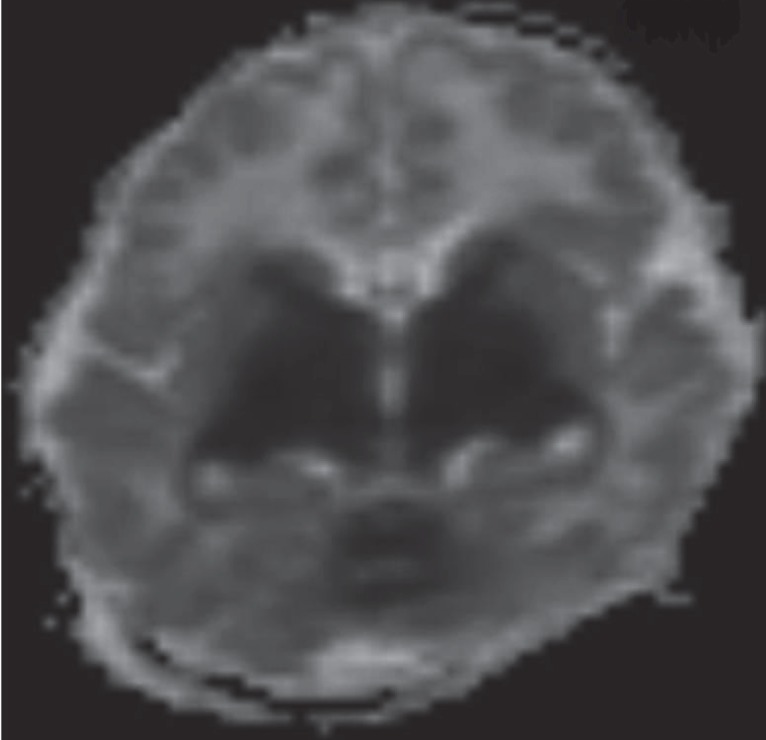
Corresponding low ADC values seen in these DWI bright areas indicative of cytotoxic oedema in this child with Maple syrup urine disease
